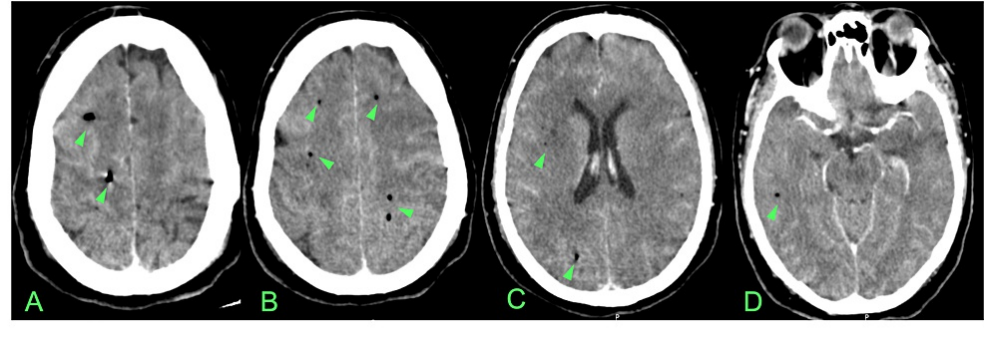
Head CT scan at admission with various axial cuts (A-D) revealing multiple small gas collections dispersed in both cerebral hemispheres (arrowheads).
